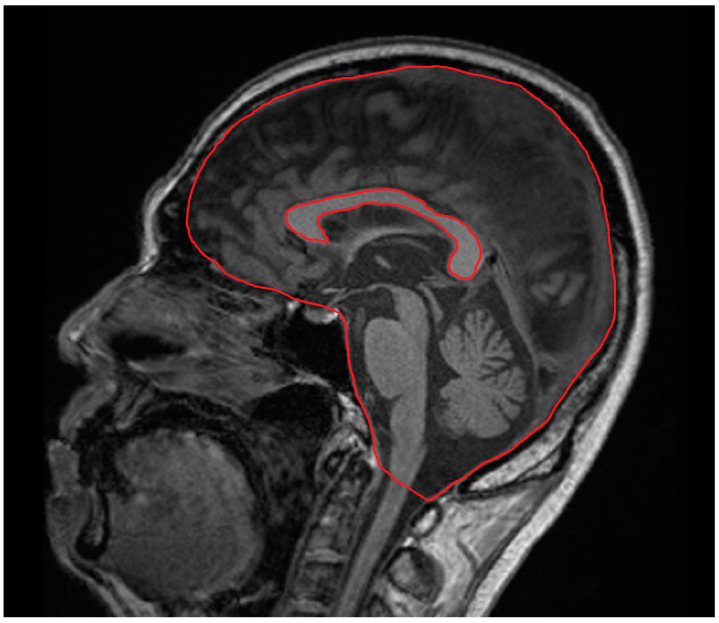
Techniques for manually measuring the corpus callosum area and intracranial skull surface area on midline T1-weighted magnetic resonance imaging sequence.
